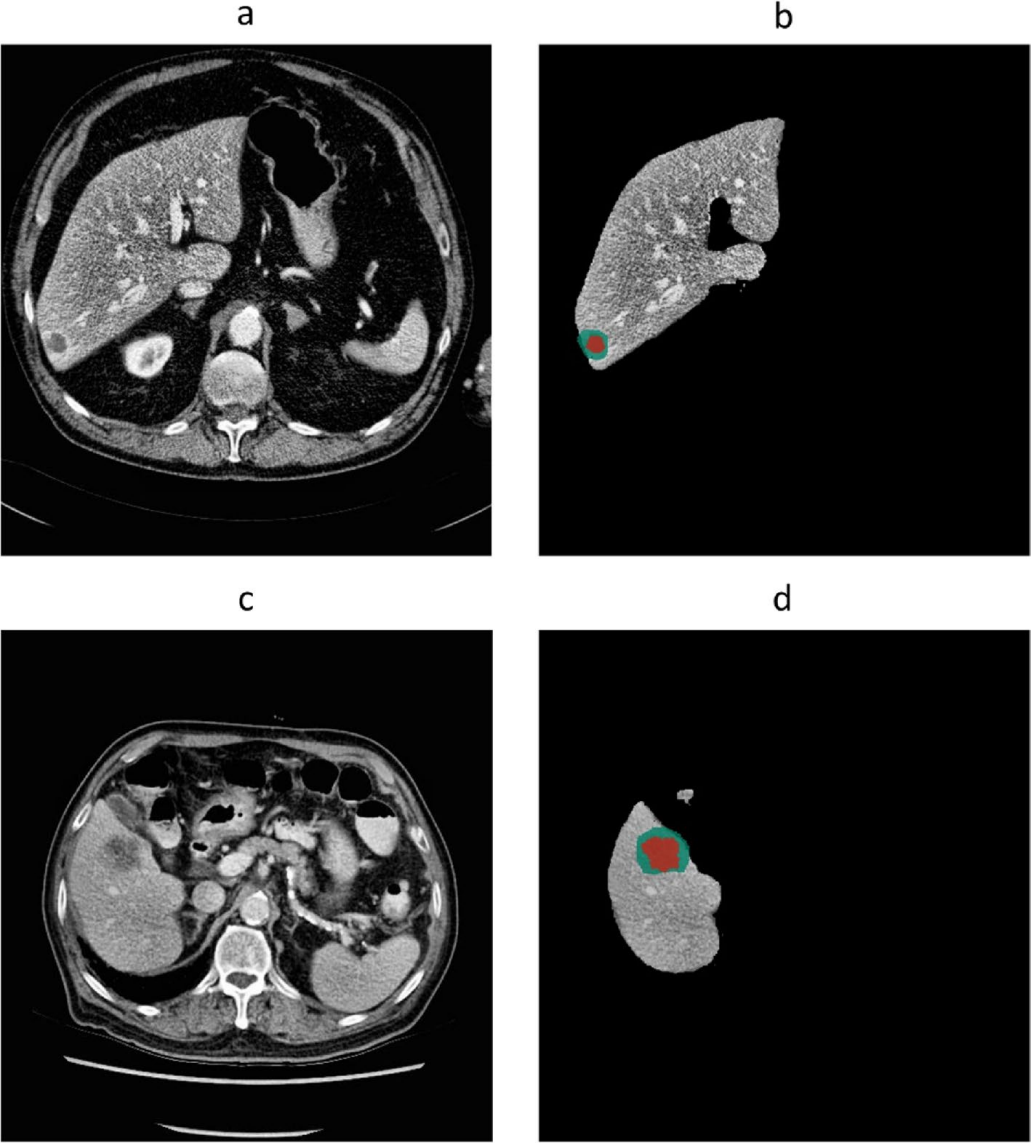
Automatic segmentations in two patients of the external validation cohort. a, c Computed tomography scans before automatic segmentation. b, d Radiologist’s tumor segmentation (dark green) and automatic tumor segmentation (red)
